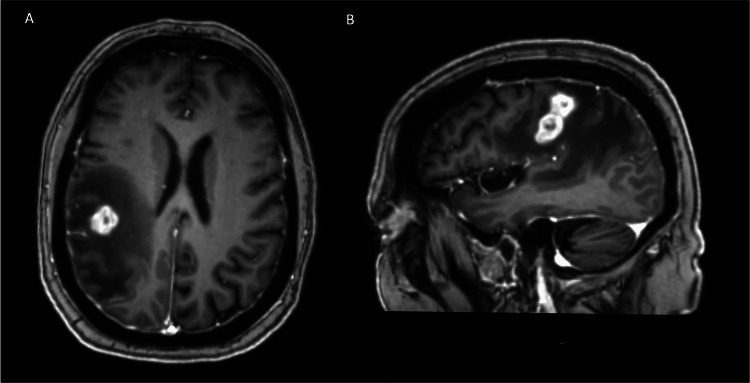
Preoperative imaging A) Axial and B) sagittal view of patient preoperative MRI
